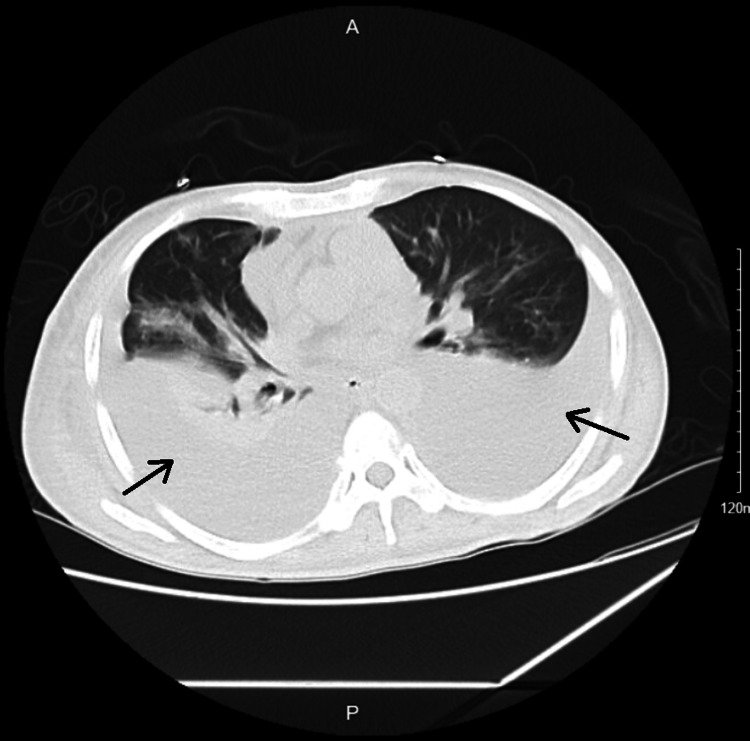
Computed tomography scan of the chest demonstrating bilateral pleural effusions (arrows)
